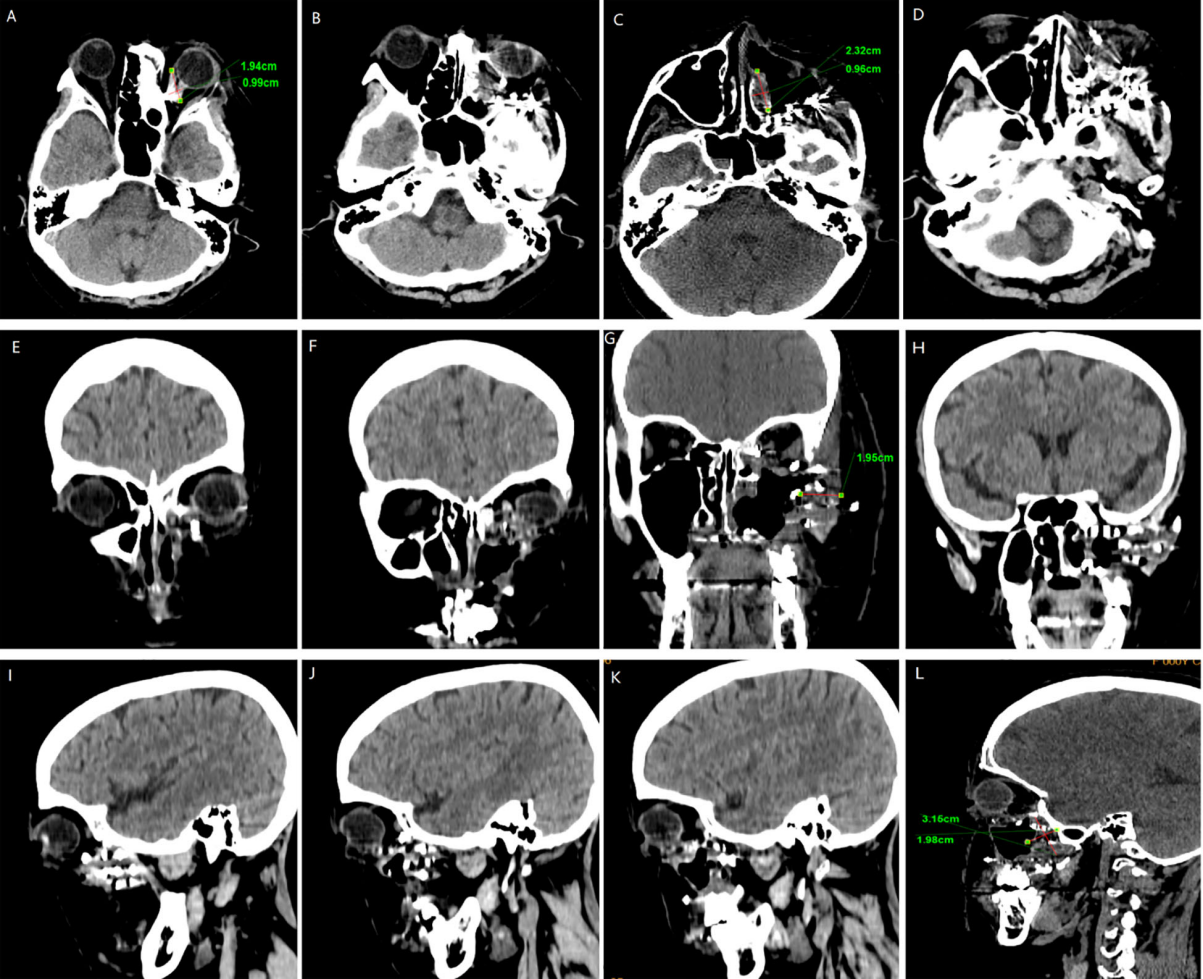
CT surveillance findings: (A–D) Axial CT image. (E–H) Coronal CT image. (I–L) Sagittal CT image (12-month follow-up).
